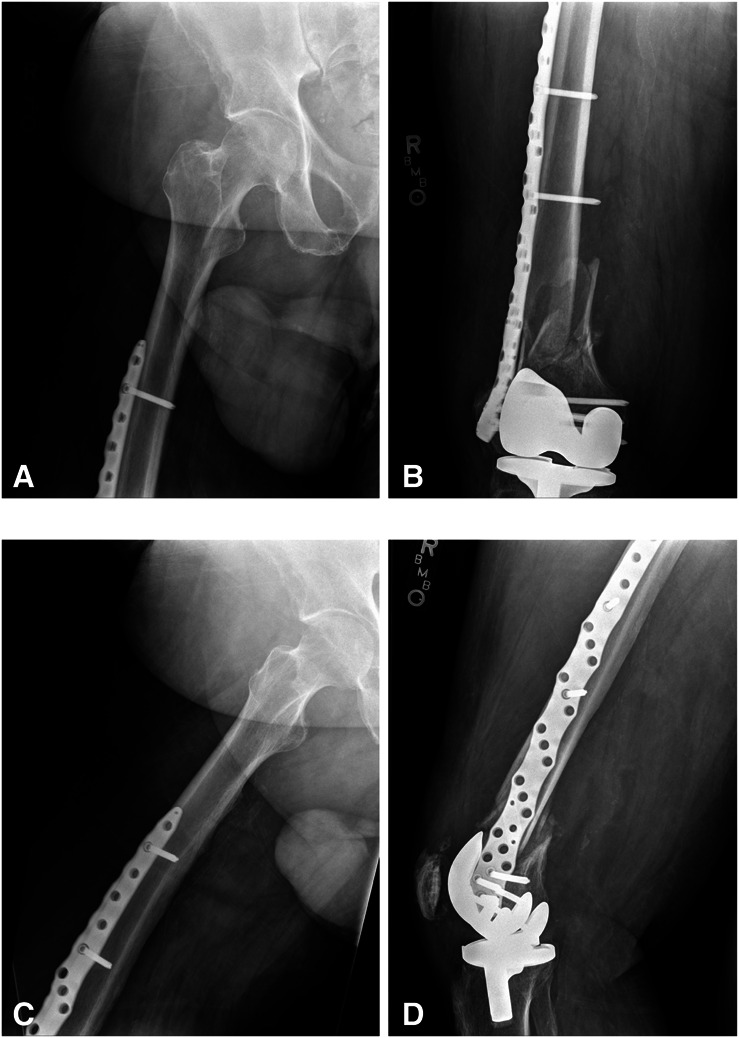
A and B, AP and (C and D) lateral radiographs of the right femur obtained 8 weeks postoperatively demonstrate early callous formation without evidence of implant failure.
